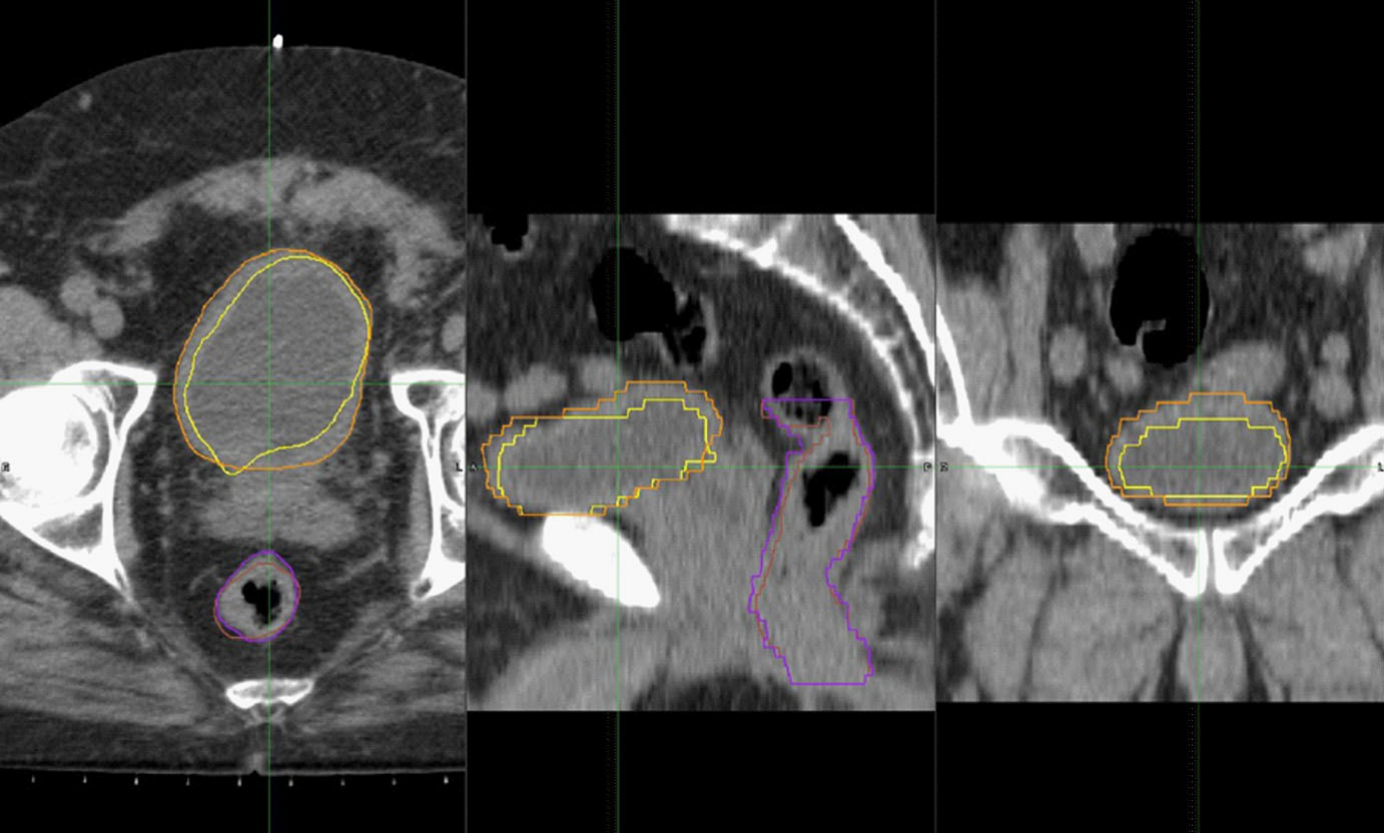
DIR Profile Propagated Contours against Manual Delineation. Fractional image guidance CT for one CTOR patient fraction with propagated contours for bladder (yellow) and rectum (brown) shown against manually delineated contours for bladder (orange) and rectum (purple) in the axial (left), sagittal (center), and coronal (right) planes.
